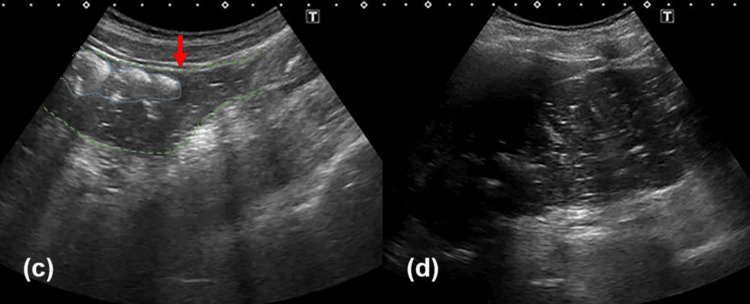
Ultrasound demonstrated colonic dilatation involving both the ascending and descending colon on day 1 (c) Ultrasound demonstrated colonic dilatation involving the sigmoid colon. The colon is outlined by the green dashed line, and the blue dashed line indicates hard stool characterized by a hyperechoic surface image. A transition from solid to liquid stool was evident at the sigmoid colon (indicated by the red arrow), supporting the diagnosis of a distal colonic obstruction. (d) Ultrasound demonstrated colonic dilatation involving the ascending colon. Intraluminal anechoic fluid collections with punctate echogenic foci were observed, consistent with liquid stool. The patient was examined in the supine position. Images were obtained using an Aplio 500 ultrasound system (Canon Medical Systems Corporation, Otawara, Japan) with a convex probe set at 3.5 MHz
